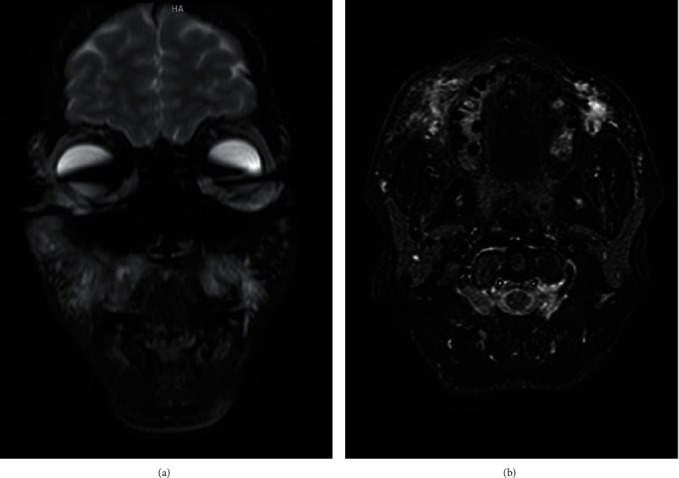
(a) MRI images revealed a subcutaneous high STIR signal fat stranding in both cheeks due to previous filler injection with adjacent mild oedema denoting a reactive inflammatory one. (b) Axial cuts displayed high signal intensity and an expansible lesion in the left cheek, with a buccal bone discontinuity.
